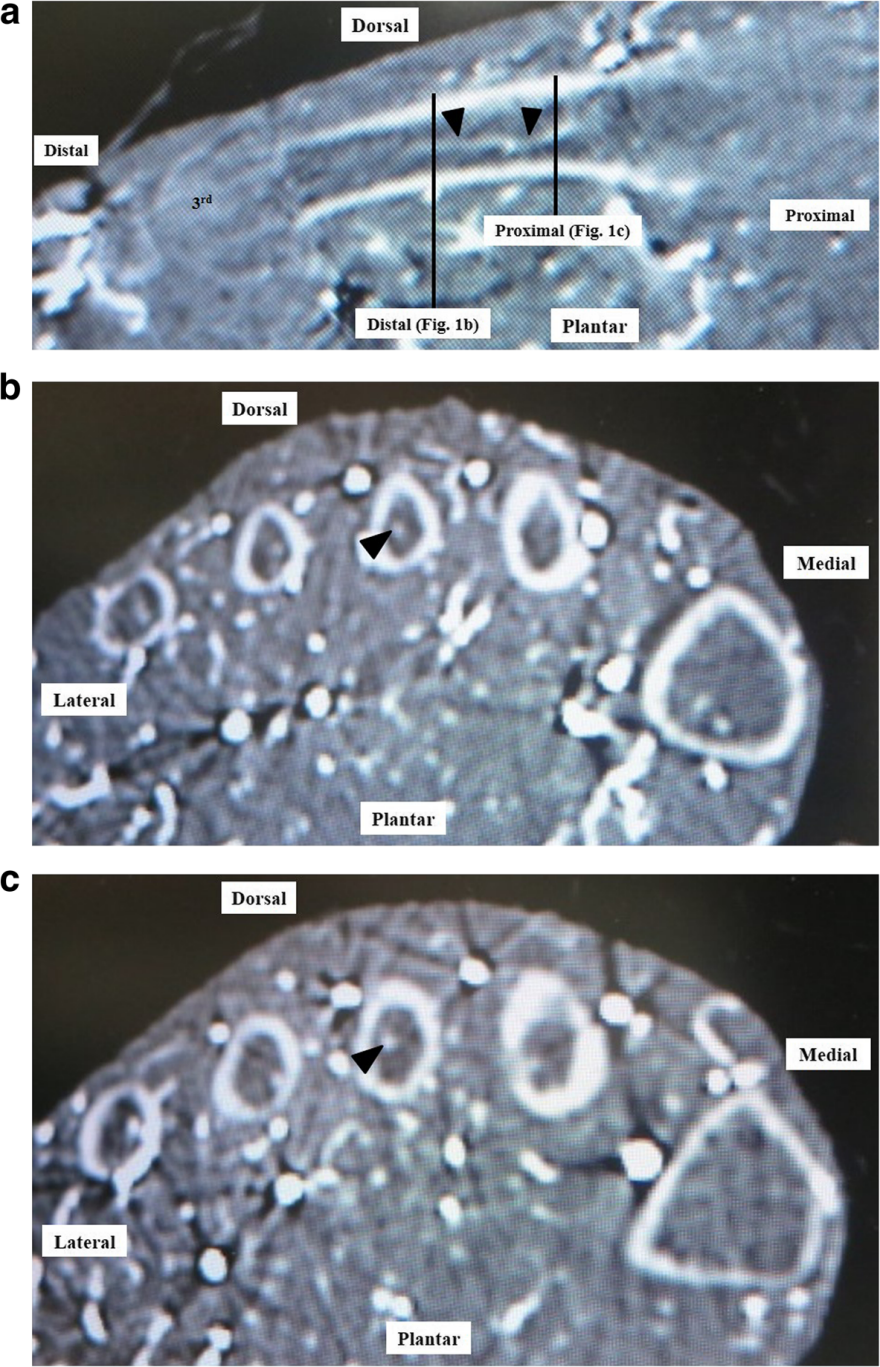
Enhanced CT images showing the nutrient artery course after entering the lesser metatarsal. The nutrient artery runs within the medullary canal distally and proximally in the sagittal plane (a) and distally (b) and proximally (c) in the axial plane. The arrow head indicates the nutrient artery
